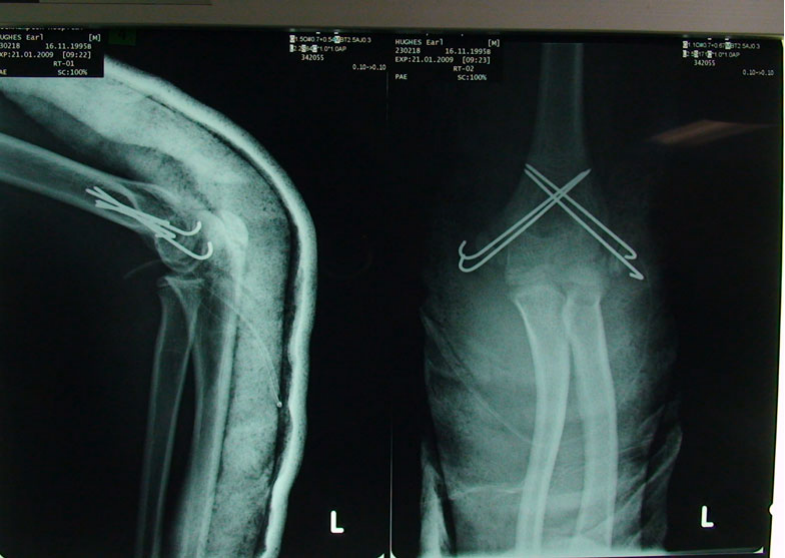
Postoperative X-ray (showing fixation with K wires).
